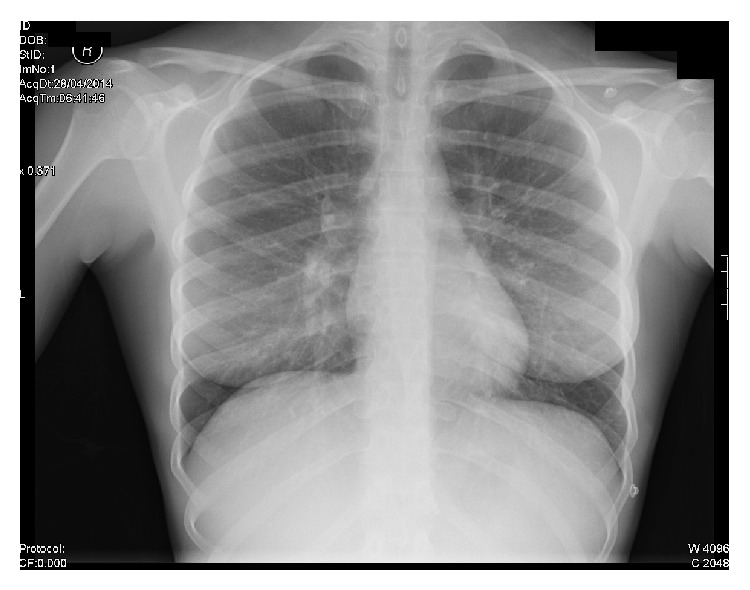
Erect chest radiograph on admission.
